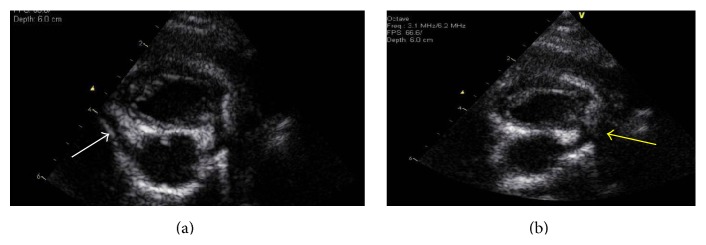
Postsurgical transthoracic echocardiographic images of the right and left coronary arteries. (a) 2D and color Doppler transthoracic image of the persistently dilated right coronary artery (white arrow). (b) 2D and color Doppler transthoracic image of the persistently dilated left main coronary artery (yellow arrow).
